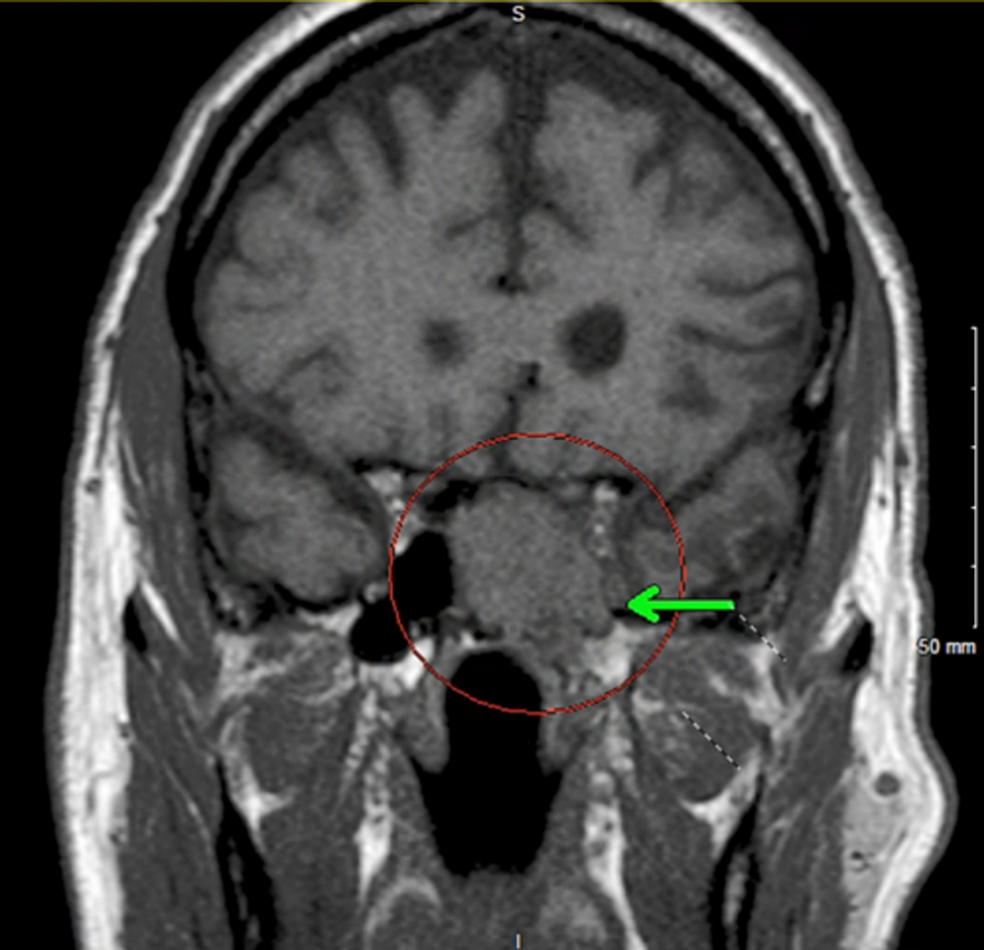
Coronal T1-weighted MRI sequence showing a mass in close proximity to the inferomedial aspect of the left optic canal (green arrow). MRI: magnetic resonance imaging
